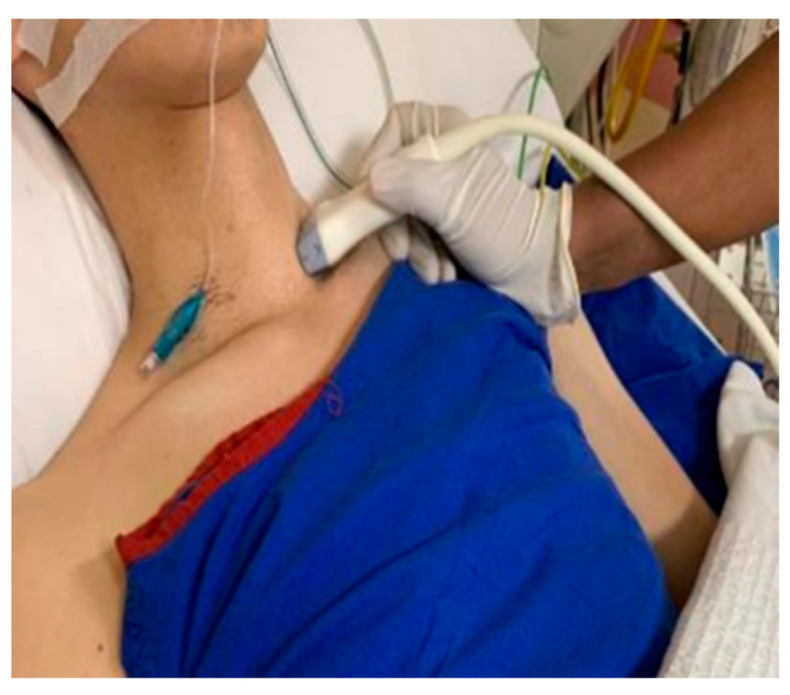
Placement of the ultrasound probe at the neck point.
